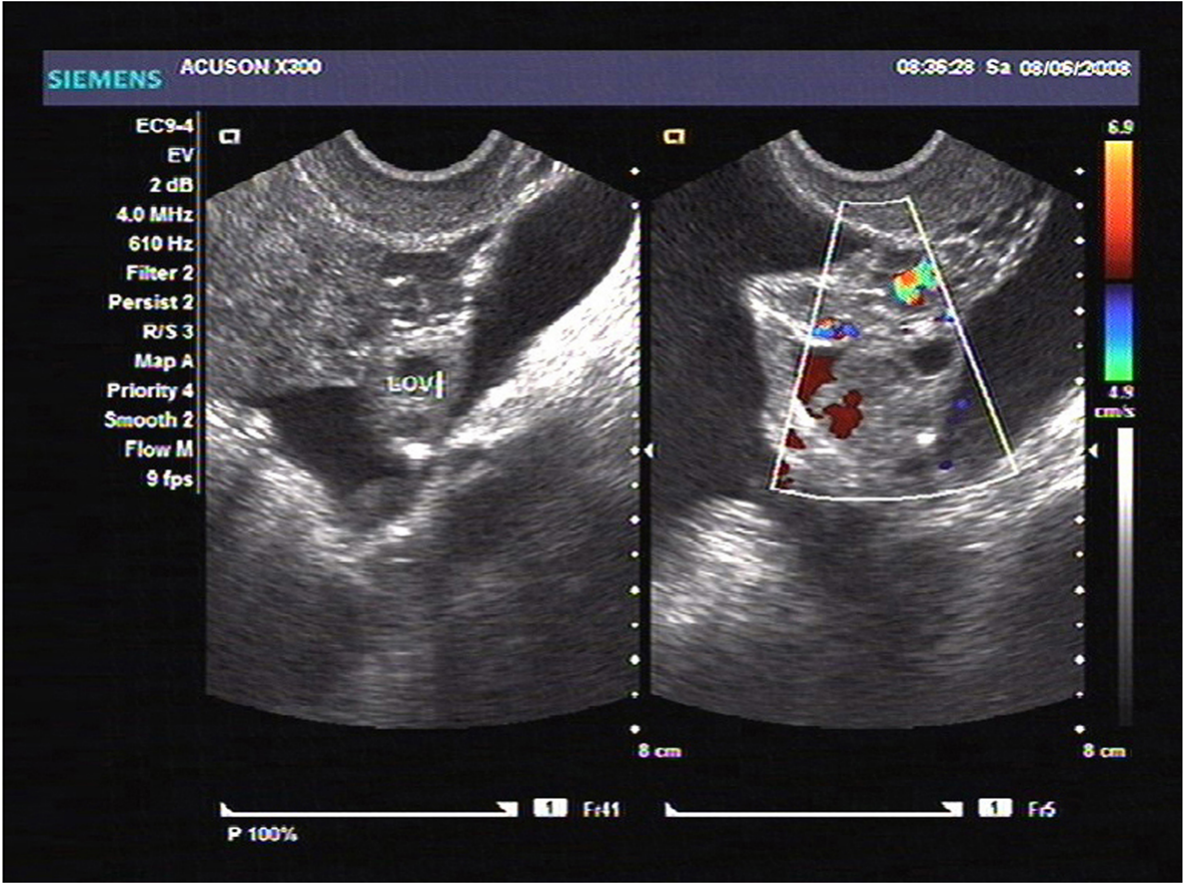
Preoperative transvaginal ultrasonography (TVUSG) showed a 5 × 4 × 5 cm mixed lump within the right adnexa. It has relatively rich blood supply signals on the circumference and inside tumor, with papillary vegetation and irregular septa, together with a small amount of pelvic fluid.
